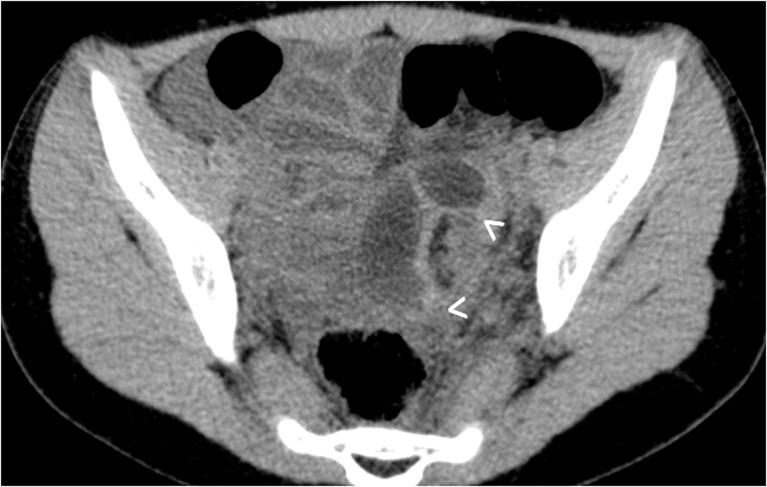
Non-enhanced CT, axial section, performed in a 12-year-old girl for unclear abdominal pain: A twisted left fallopian tube can be visualised (*arrowheads*)
